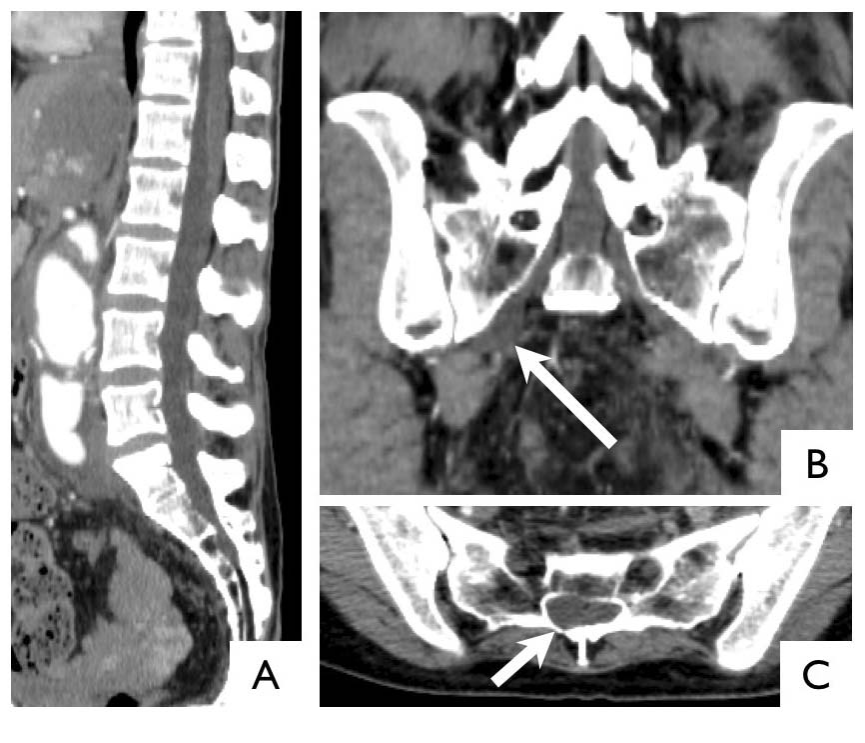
CT images of 46-year-old female with Loeys-Dietz syndrome. Sagittal image of the normal dura (A). Coronal image of right lateral meningocele (arrow) (B). Axial image at S1 shows asymmetric dilatation of the dura (arrow) (C). In this case, visual inspection could detect dural ectasia, but quantitative evaluation could not.
